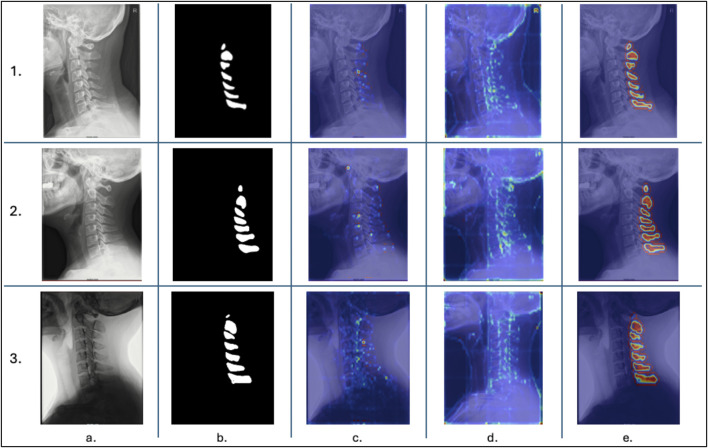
Transformer Attention Maps for ViT-B. Attention maps generated for three test cases; two with good (1, 2) and one with bad metrics and predicted masks (3). (a) is the original radiograph, (b) is the hybrid model predicted mask, (c) is the query centric map, (d) represents the attention-rollout map, and (e) depicts the Grad-CAM map.
